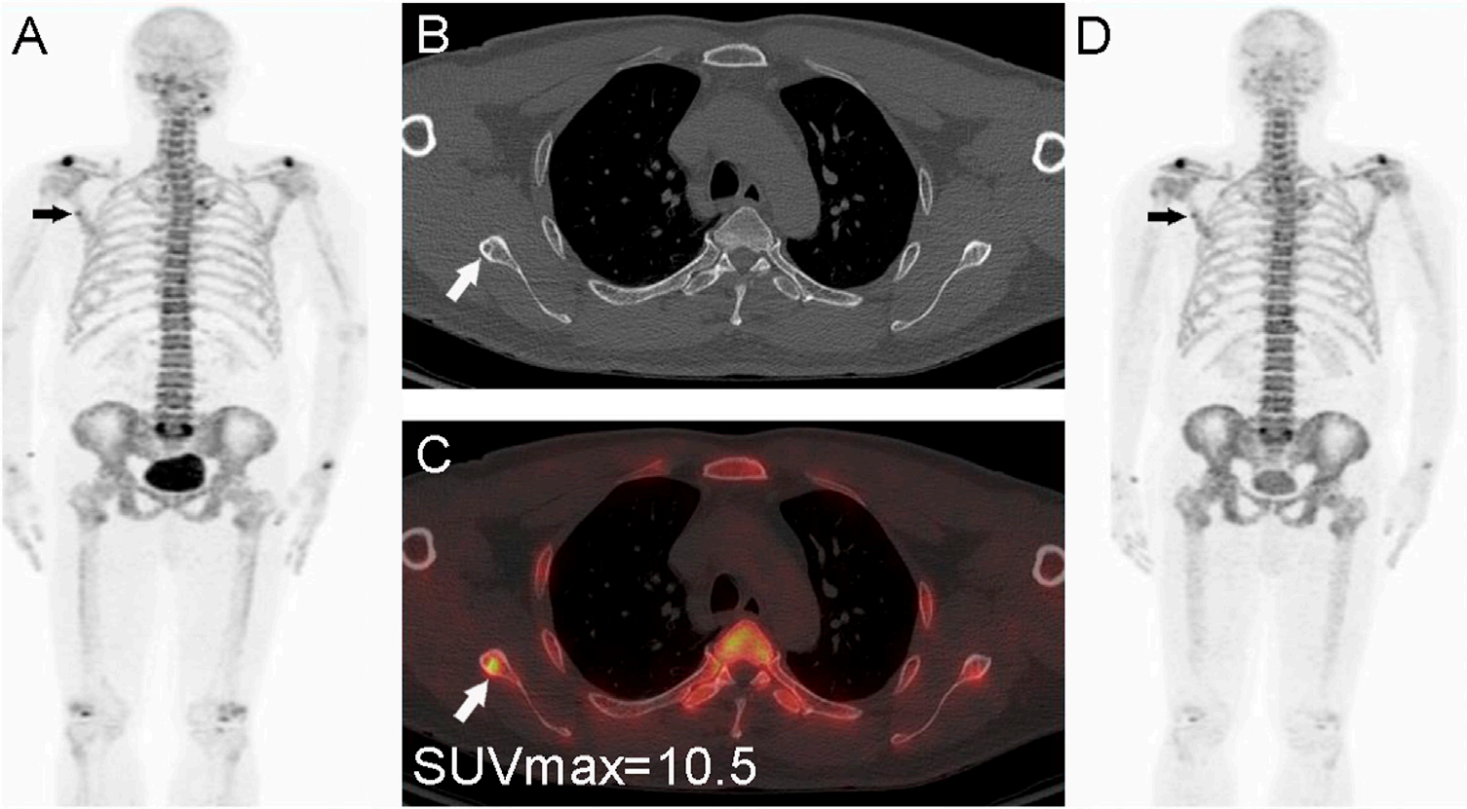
Nasopharyngeal carcinoma (T2N2M0) in a 56-year-old male patient. The whole-body PET MIP image (A), arrow shows a focal mild abnormal ^18^F-NaF uptake in the right scapula, CT (B), small arrow shows a small sclerotic border, PET/CT image (C) shows a localized ^18^F-NaF uptake, and measurement of the lesion with SUVmax = 10.5 < 12.5. The lesion was first considered benign based on the CT findings and the degree of metabolism. A follow-up PET/CT (D), arrow at 1 year showed no metabolic changes and thus confirming the benign nature.
